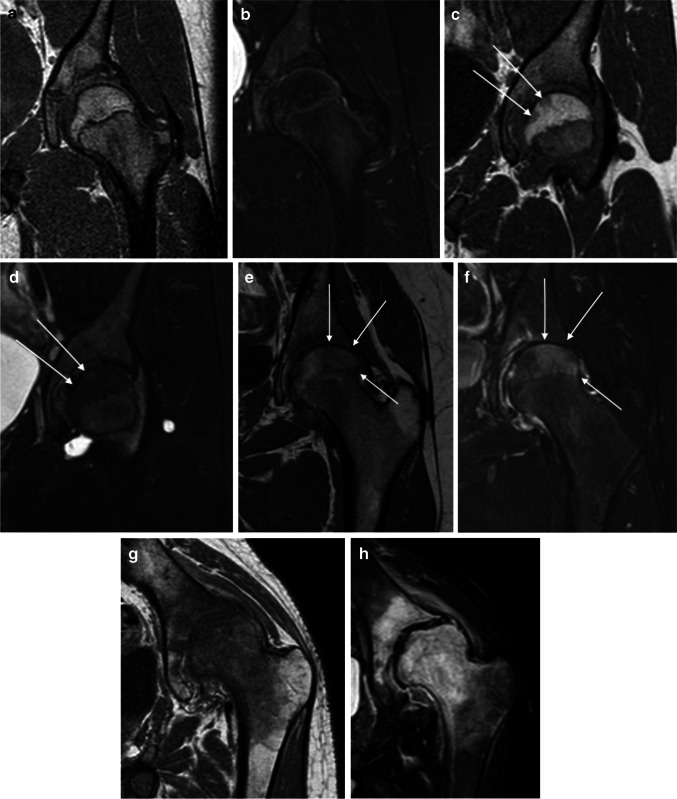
Femoral head bone marrow edema demonstrated on MRI of the left hip in four pairs of images from four children with juvenile idiopathic arthritis (JIA) across different severity levels. Bone marrow edema was defined as hypointense areas on T1-W sequences with corresponding hyperintense areas on fat-saturated T2-W sequences in the bone marrow. All images are demonstrated using coronal three-dimensional (3-D) T1-weighted turbo spin-echo (a, c, e, g) and fat-saturated T2-weighted turbo spin echo (b, d, f, h) MRI sequences. a, b No visible bone marrow edema (score 0) in a 13-year-old boy. c, d Two focal areas of bone marrow edema (less than 33% of the bone volume, *arrows*) (score 1) in a 11-year-old girl. e, g Large area of bone marrow edema (between 33 and 66% of the bone volume, *arrows*) (score 2) in a 14-year-old girl. g, h Widespread bone marrow edema (almost 100% of the bone volume) (score 3) in a 15-year-old boy
